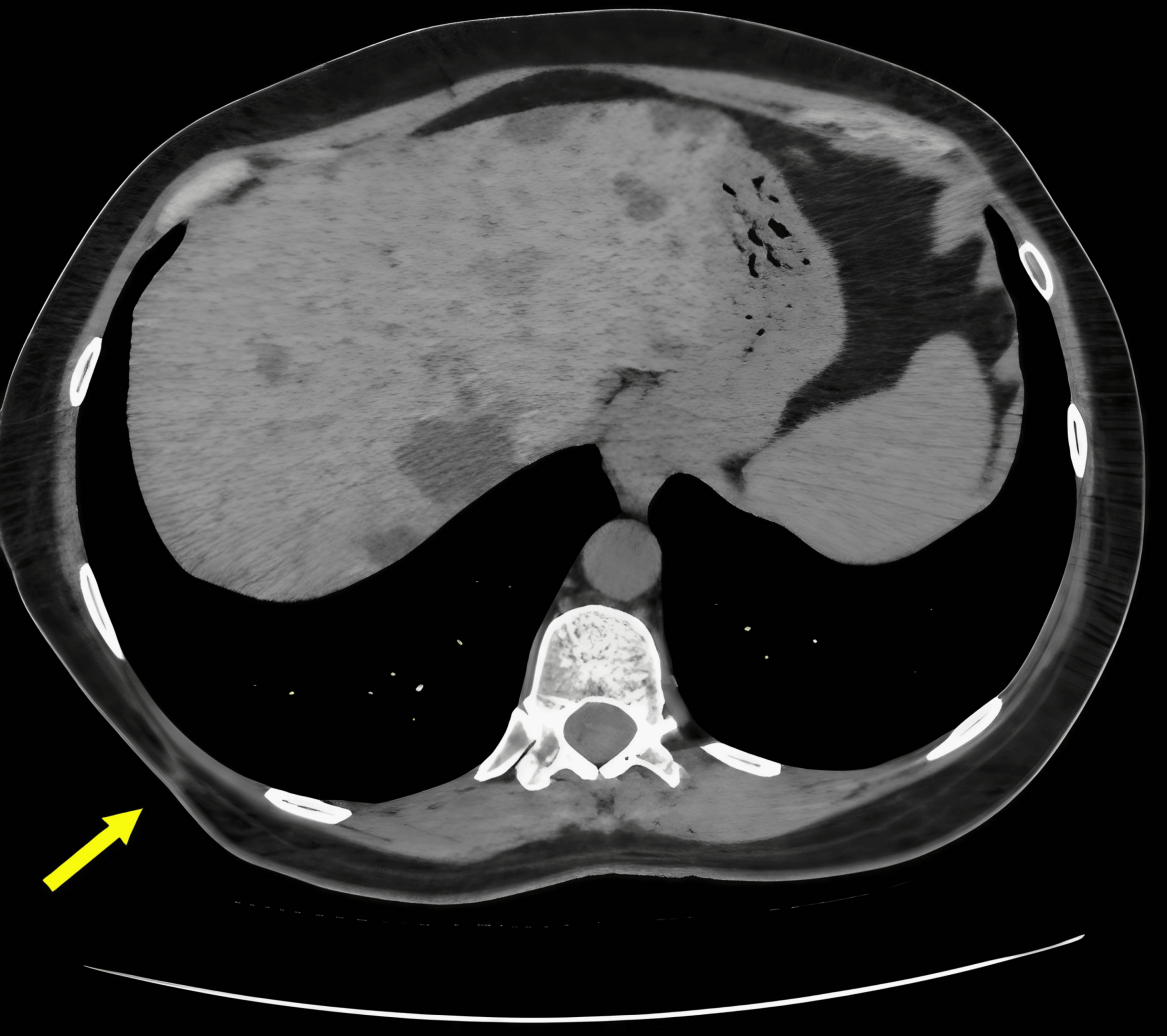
Axial non-contrast CT from February 2025 demonstrates postoperative changes with the absence of the mass and no evidence of local recurrence (yellow arrow).
